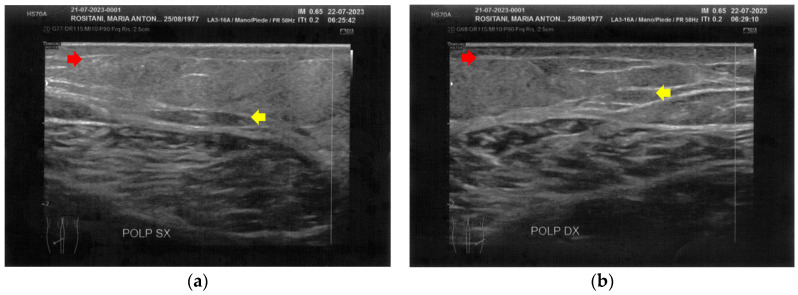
Ultrasonography of the calves at T1. (a) The left side presents a clear improvement in both the cicatricial fibrosis (red arrow) and the reorganization of the different structural layers (yellow arrow). The muscular structure appears clearly defined, with a net improvement concerning the initial condition; (b) The right side presents the same treatment effects noted for the left side. On this side, the muscular structure was less compromised than that of the left side. The treatment acts on the connective tissue, softening the structure.
